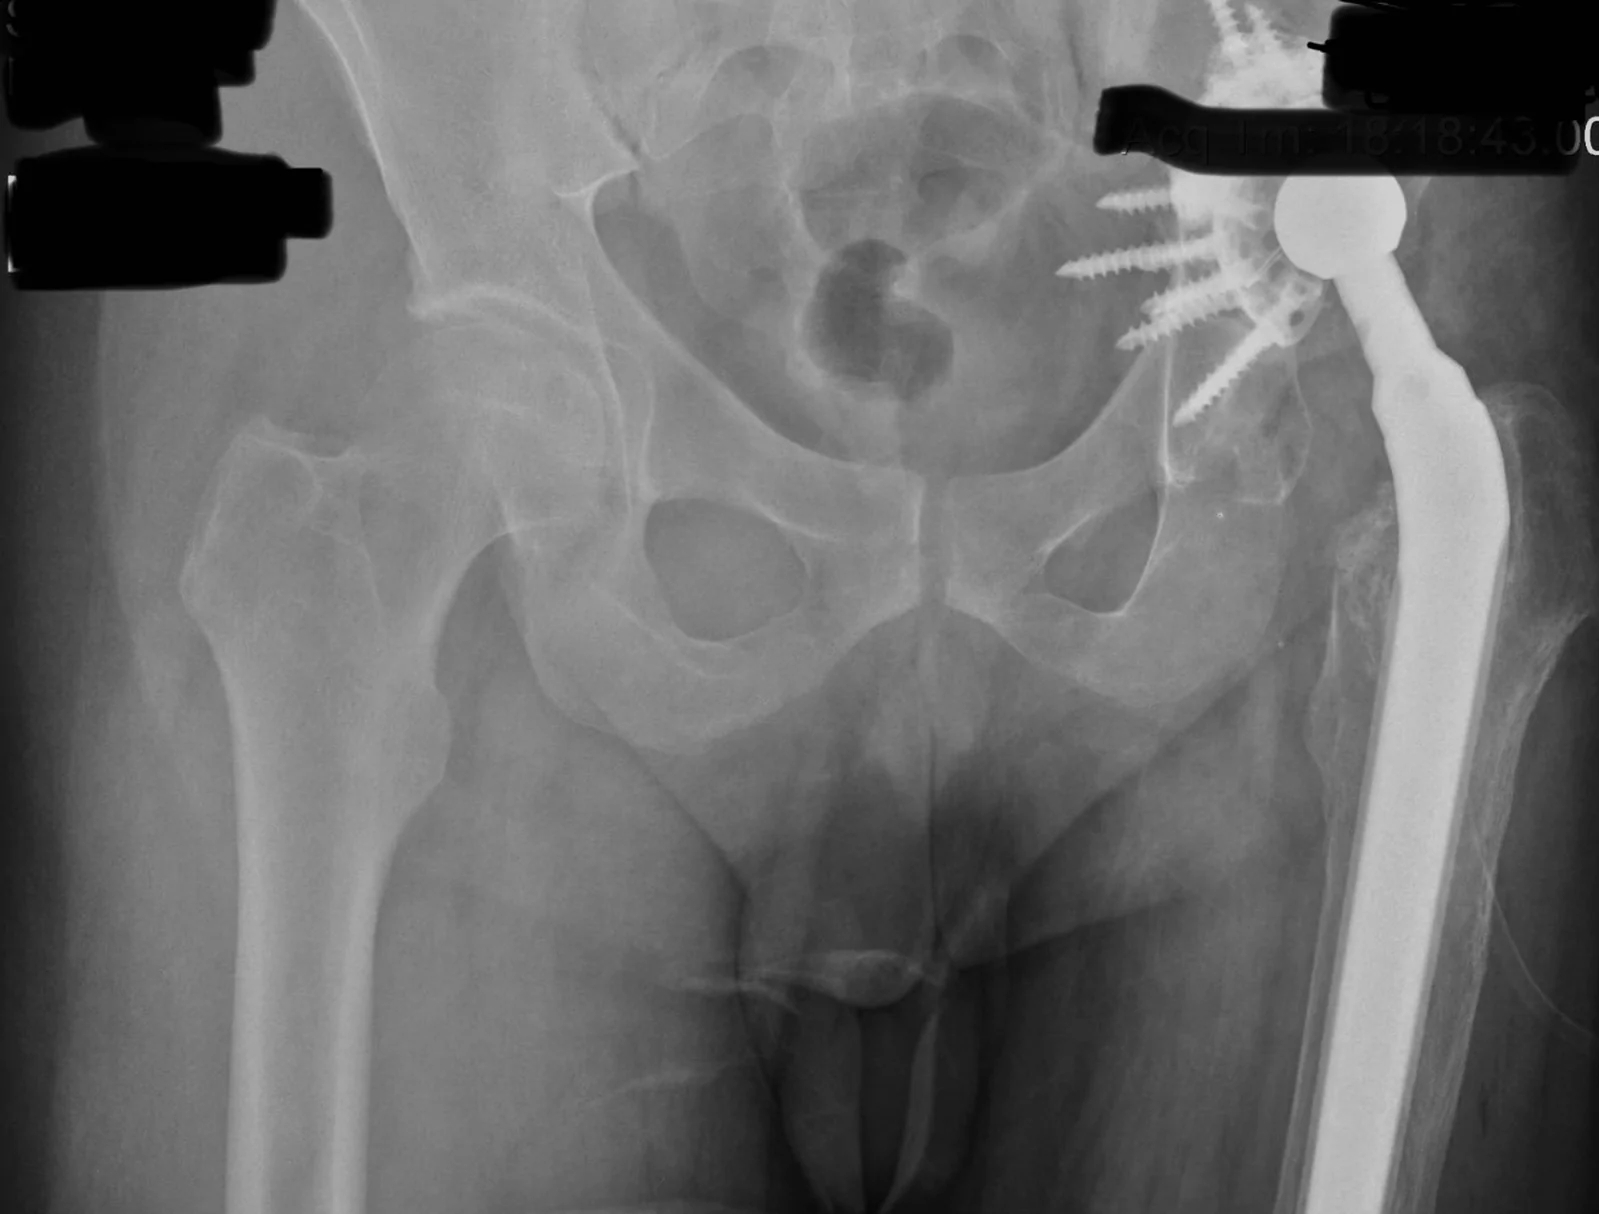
Postoperative radiographic findings. Postoperative radiograph demonstrating left acetabular reconstruction, restoring the anatomical center of rotation. Porous titanium augments and bone graft fill the vault defect, with rigid multi-screw transacetabular fixation and correction of leg-length discrepancy.
